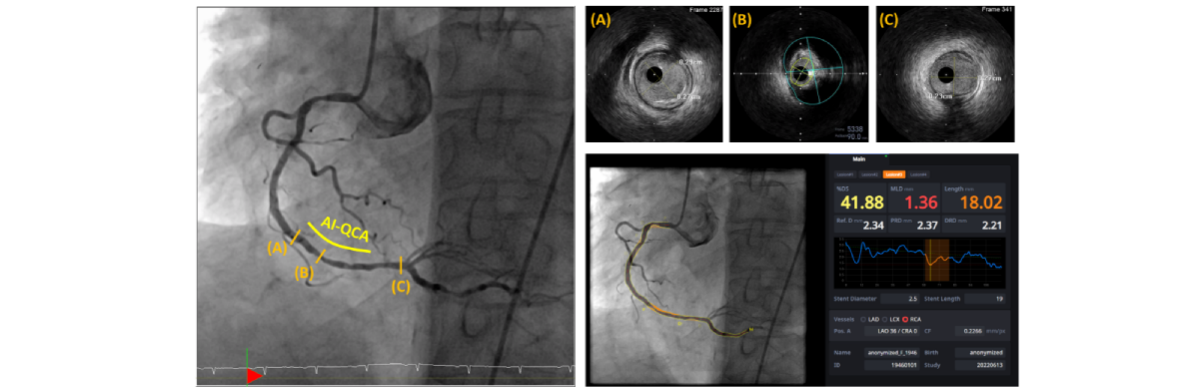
A representative case in which artificial intelligence–based quantitative coronary angiography (AI-QCA) measured lesion length shorter than intravascular ultrasound (IVUS). AI-QCA identified mild atherosclerotic lesion at distal as normal. %DS: percent diameter stenosis; AI-QCA: artificial intelligence–based quantitative coronary angiography; DRD: distal reference diameter; LAD: left anterior descending artery; LCX: left circumflex artery; MLD: minimal luminal diameter; PRD: proximal reference diameter; RCA: right coronary artery; Ref.D: reference diameter.
